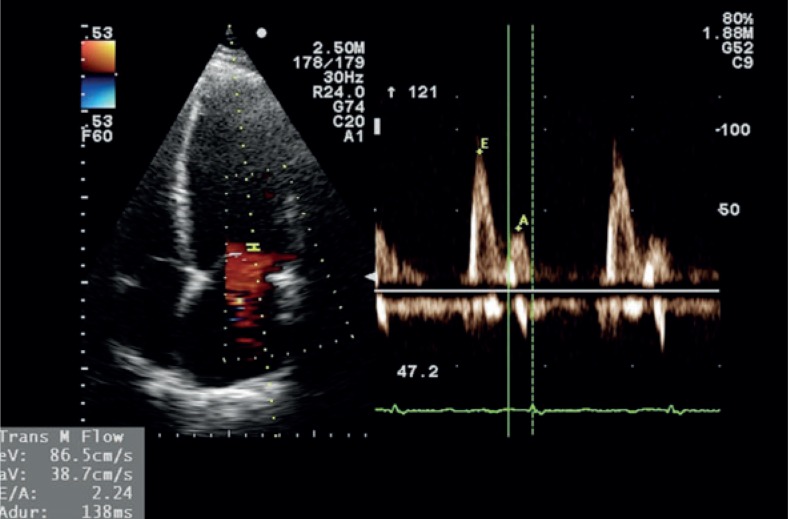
Pulsed wave Doppler measurement of flow velocities through the mitral valve annulus showing normal left ventricular filling pattern. (E = early diastolic flow velocity, A = atrial contraction flow velocity).
